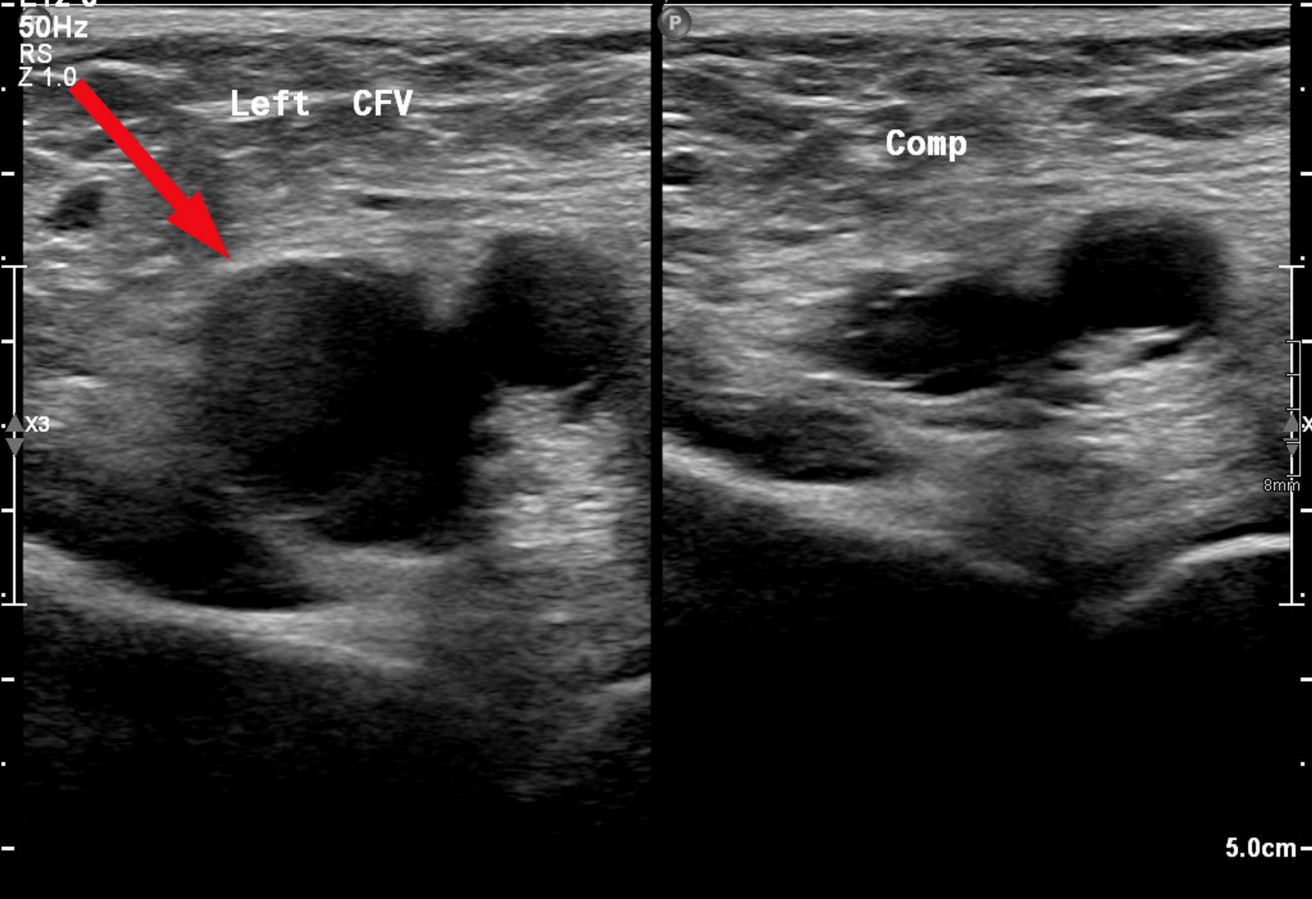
Left common femoral vein (CFV) with thrombus within on ultrasound compression venography before and after compression
